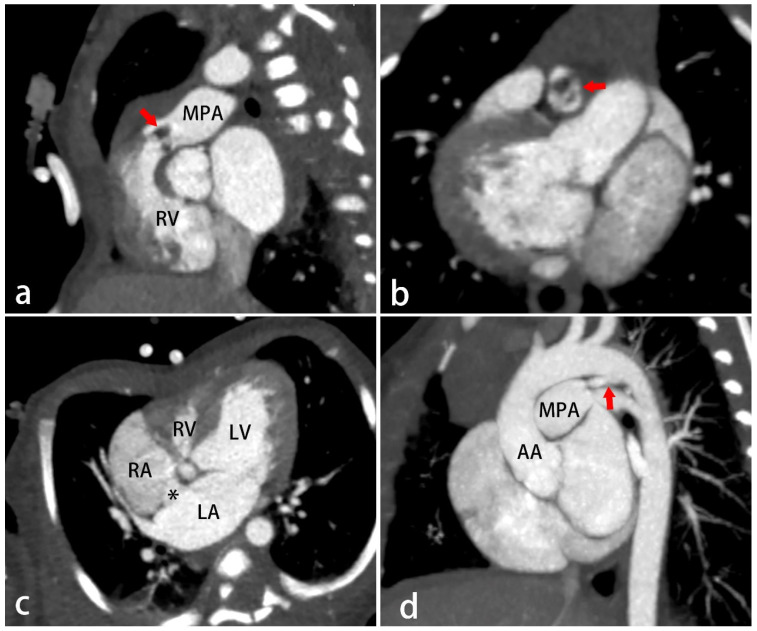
A 7-day-old boy with pulmonary valve dysplasia. CT angiography oblique images show a bicuspid pulmonary valve with irregular thickening of the leaflets and stenosis of the valve orifice ((a,b), red arrow). Four-chamber view demonstrates tricuspid atresia, hypoplastic right ventricle, and atrial septal defect ((c), black asterisk). Maximum intensity projection (MIP) image shows the presence of PDA ((d), red arrow). LV, left ventricle; LA, left atrium; RV, right ventricle; RA, right atrium; AA, ascending aorta; MPA, main pulmonary artery.
